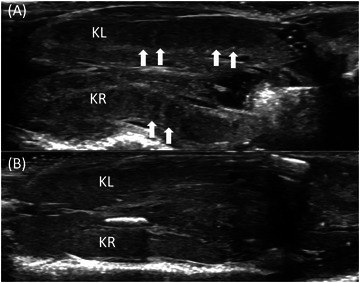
Comparison between male and female kidneys of veiled chameleons in ultrasound. A 12 MHz linear transducer was used in each case. A, Left (KL) and right (KR) kidney of a male veiled chameleon. The sexual complexes appear as hypoechogenic striations (arrow above). B, Left (KL) and right (KR) kidney of a female veiled chameleon. The kidney tissue is homogeneous.
